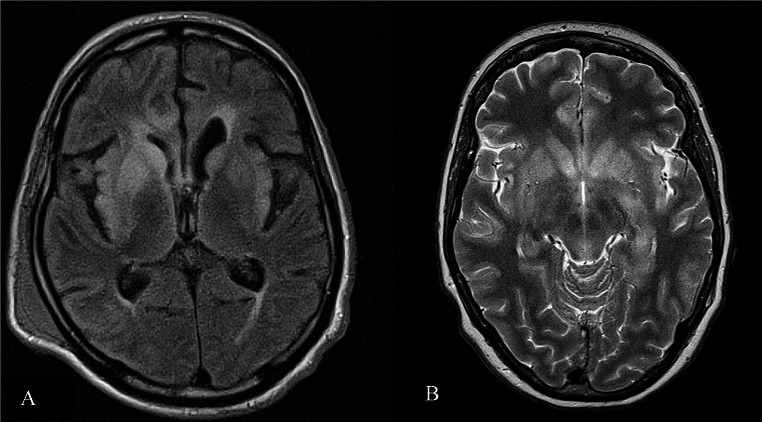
Exemplified MRI images of BoDV-1 and VSBV-1 encephalitis study cases. A. Bilateral edema in the basal ganglia and the insula pronounced on the right side in BoDV-1 encephalitis (FLAIR). B. Bilateral edema in the basal ganglia and in limbic structures (insula, hippocampus, anterior cingulate) in VSBV-1 encephalitis (T2-weighted)
